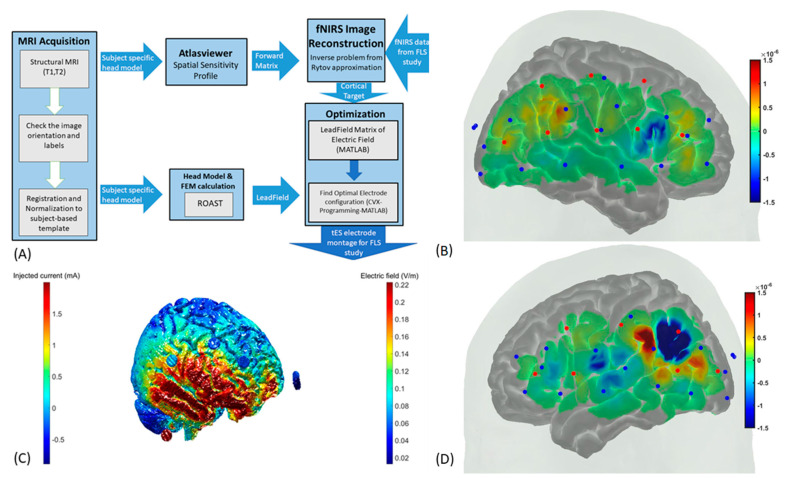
Neuroimaging-guided transcranial electrical stimulation. (A) An individualized head model can be created from structural MRI in SPM software (https://www.fil.ion.ucl.ac.uk/spm/: accessed on 1 June 2021) that can be used in AtlasViewer and ROAST to create a subject-specific forward matrix and lead field, respectively. AtlasViewer uses the forward matrix to estimate the cortical activation from task-related fNIRS data, which can be used as the cortical target for the electrode optimization in ROAST based on the lead field. (B) Right hemisphere cortical activation from AtlasViewer during the execution of a complex motor task (red dots show the sources and blue dots show the detectors) from Li et al. [43]. (C) Right hemisphere cortical activation during the execution of a complex motor task (from Li et al. [43]) was targeted with the ROAST-optimized electrode montage as shown by the electric field distribution (V/m). (D) Left hemisphere cortical activation from AtlasViewer during the execution of a complex motor task from Li et al. [43].
